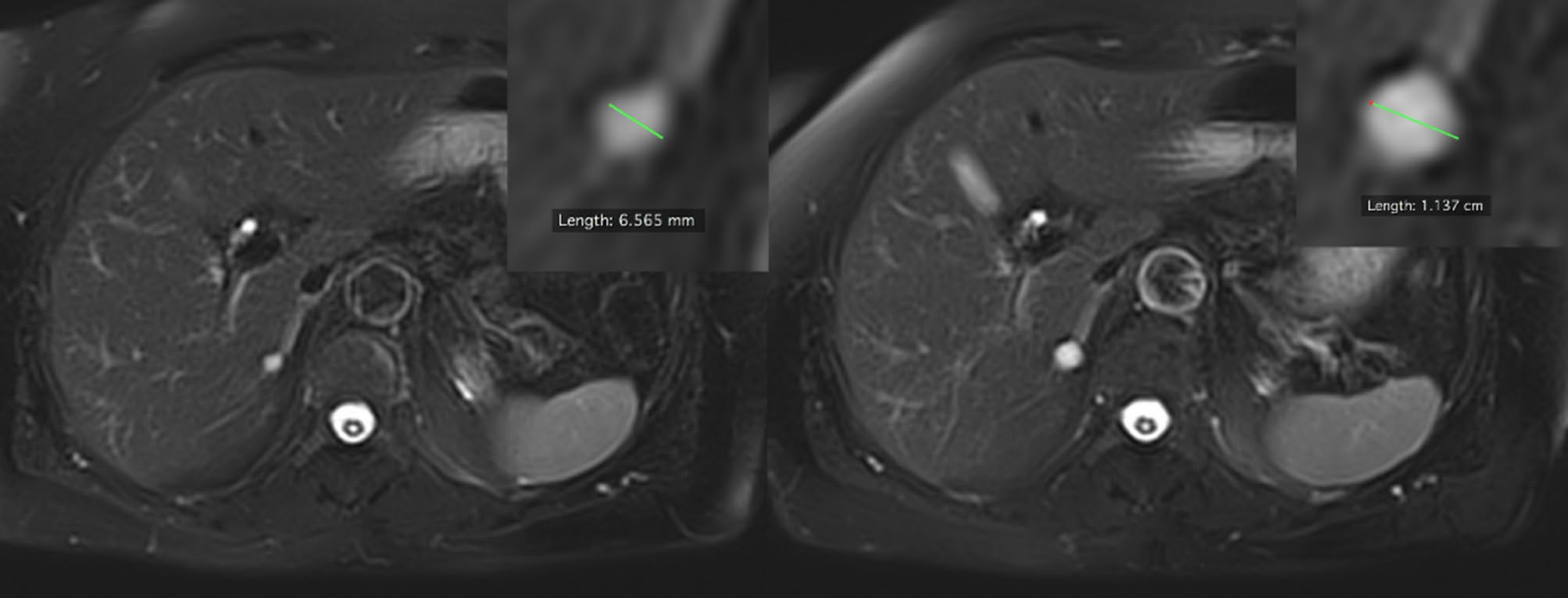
Case with a cyst at baseline and follow-up in segment 6 of the liver on axial T2-weighted TSE sequence. The enlarged segments in the right upper corner of both images depict an example of a cyst measurement. Its size changed from 6.6 mm to 11.4 mm.
